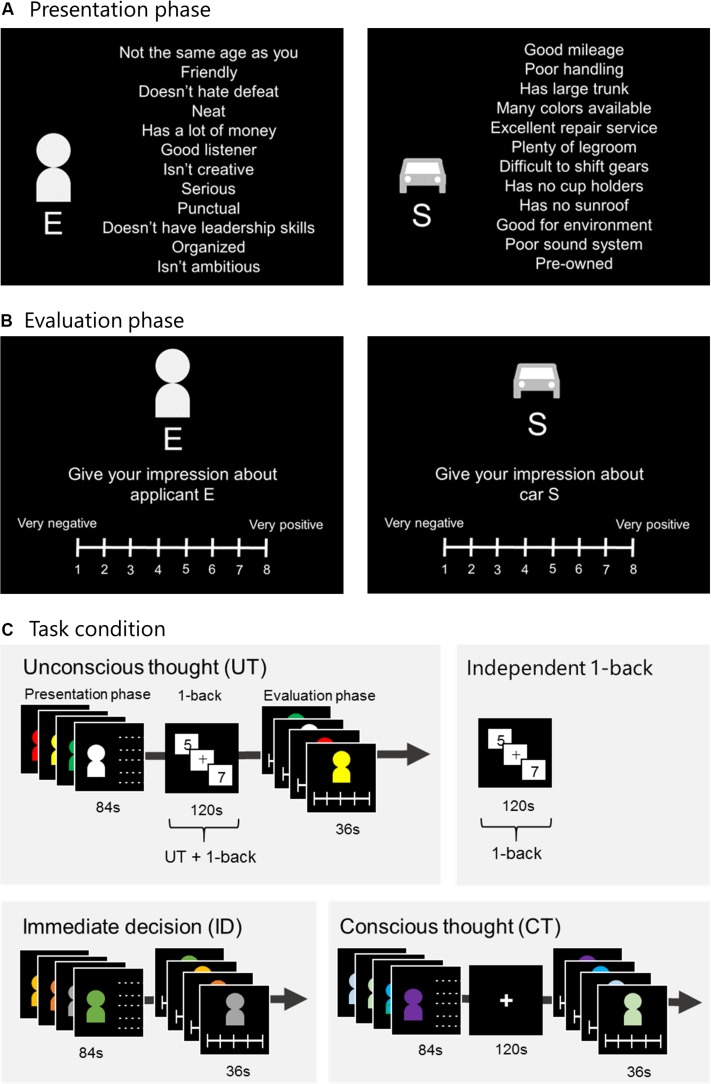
Experimental task. (A) Presentation phase: Alternatives E–H were presented in a random order for 21 s each. (B) Evaluation phase: Participants rated each alternative within 9 s using an 8-point Likert scale. (C) Under UT conditions, participants engaged in a 1-back task for 120 s between the presentation and evaluation phases. Under ID conditions, the evaluation phase was immediately followed by the presentation phase. Under CT conditions, after presenting the alternatives, participants were given 120 s to think carefully. The independent 1-back task served as the control task for UT, and participants were instructed to engage in the task. UT, unconscious thought; CT, conscious thought; ID, immediate decision.
